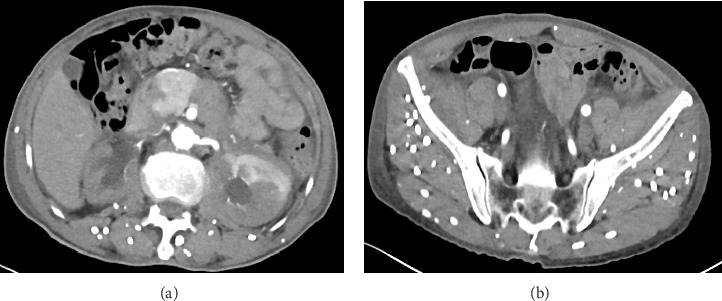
Abdominal CT scan showing (a) retroperitoneal fibrosis encasing the ureters, and (b) multiple calcified lesions consistent with cysticercosis.
